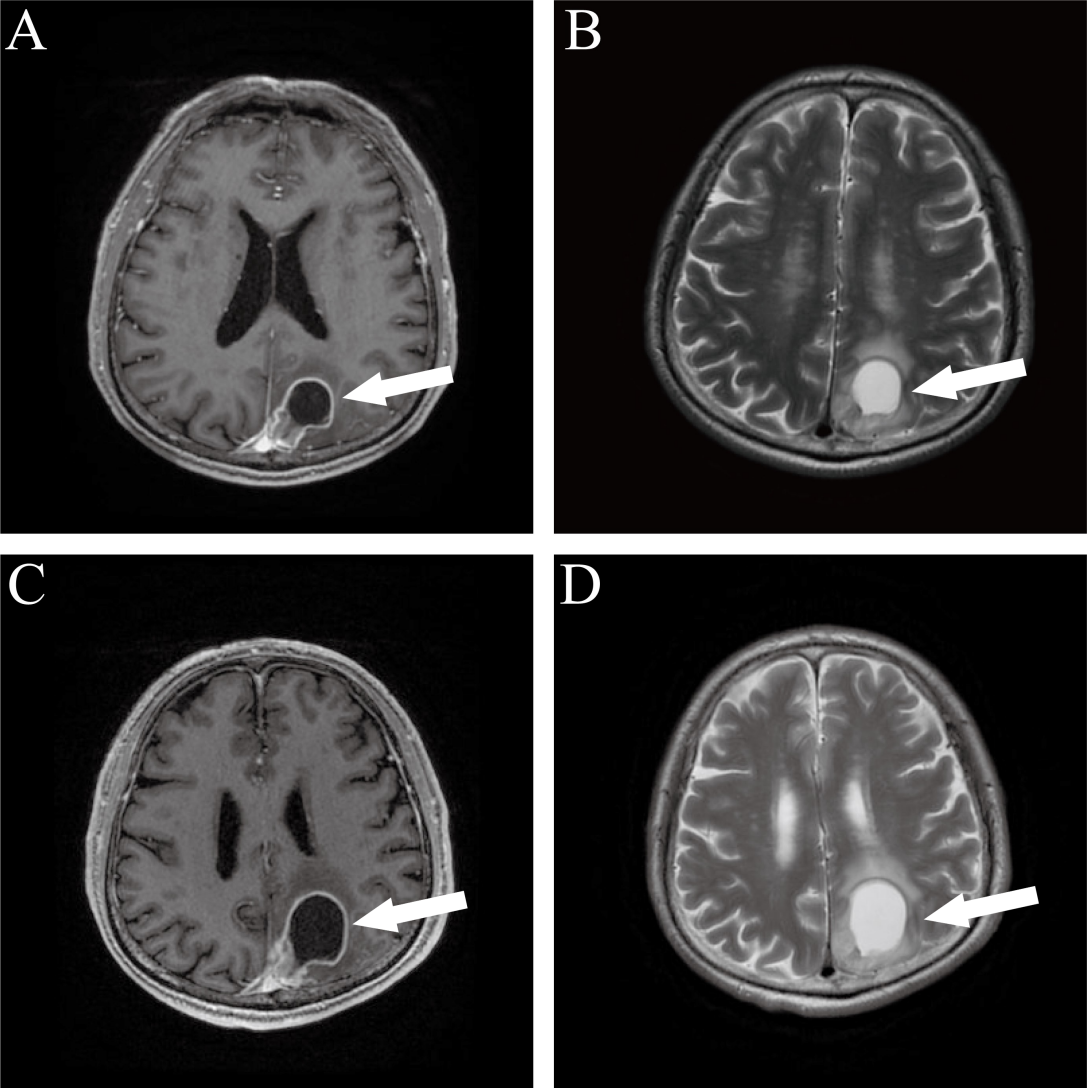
Serial brain MRI follow-up. (A) Contrast-enhanced axial T1-weighted imaging (T1WI+C) reveals a left parietal cystic-solid mass with ring enhancement of the cyst wall and surrounding edema. (B) Axial T2-weighted imaging (T2WI) shows uniform high signal intensity within the cystic region and slightly hyperintense perilesional edema. (C, D) Followup imaging approximately one month later. (C) T1WI+C demonstrates lesion enlargement compared to previous scan, with obscured boundaries adjacent to the superior sagittal sinus. (D) Axial T2WI reveals newly developed patchy, slightly prolonged T2 signal in the pons with ill-defined margins.
